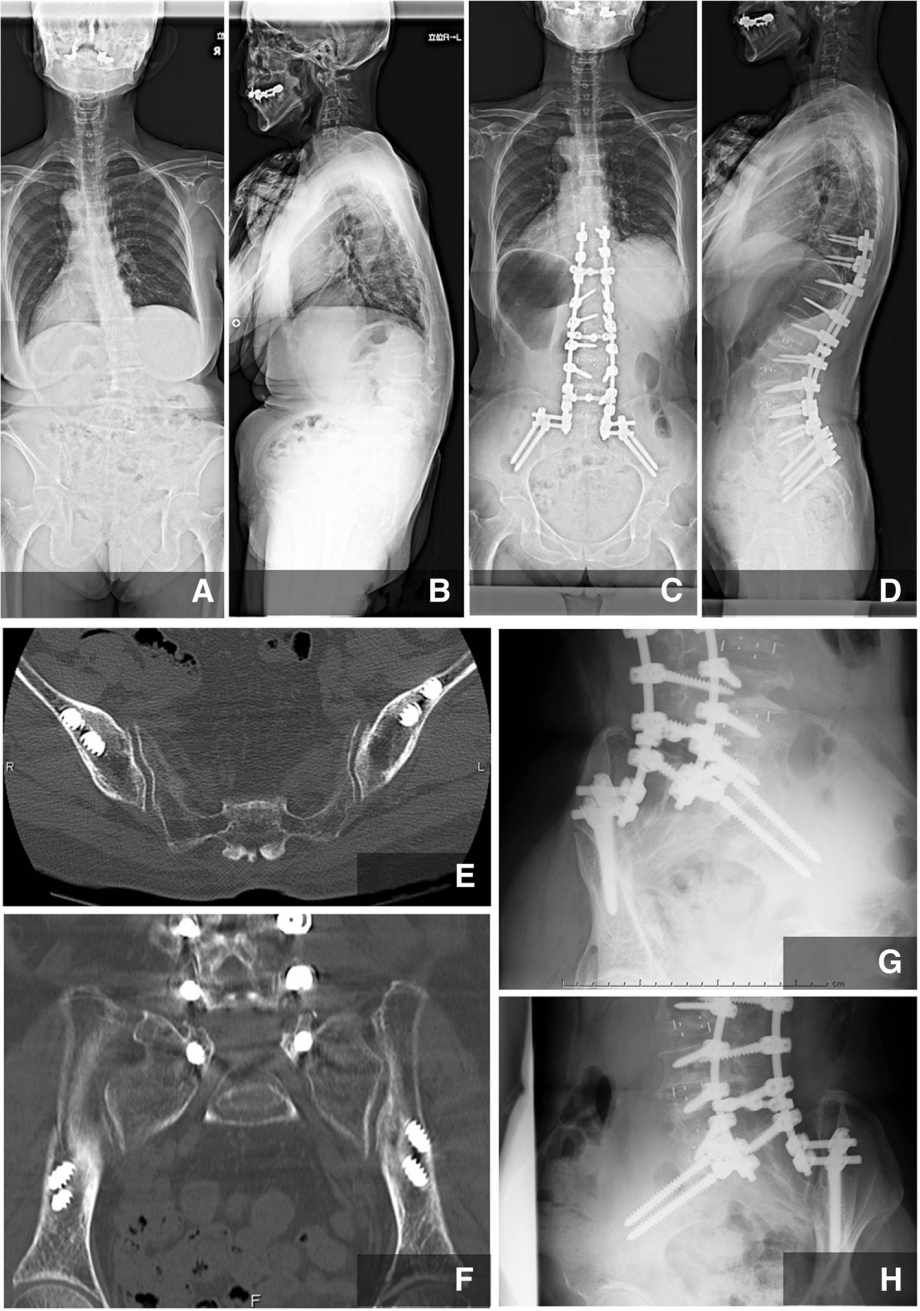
Case presentation. A 72-year-old female patient had adult spinal kyphosis and showed a postural imbalance, such as leaning forward or to the left side when walking and standing, resulting in claudication within 2 min. She underwent surgery including LIF at L2–3, 3–4, and 4–5 disc levels and posterior lumbar interbody fusion at L5-S1, and posterior corrective fusion from T10 to the ilium. a Preoperative A-P view, b preoperative lateral view, c postoperative A-P view, d postoperative lateral view, e postoperative CT axial view, f postoperative CT coronal view, g, h postoperative oblique views
